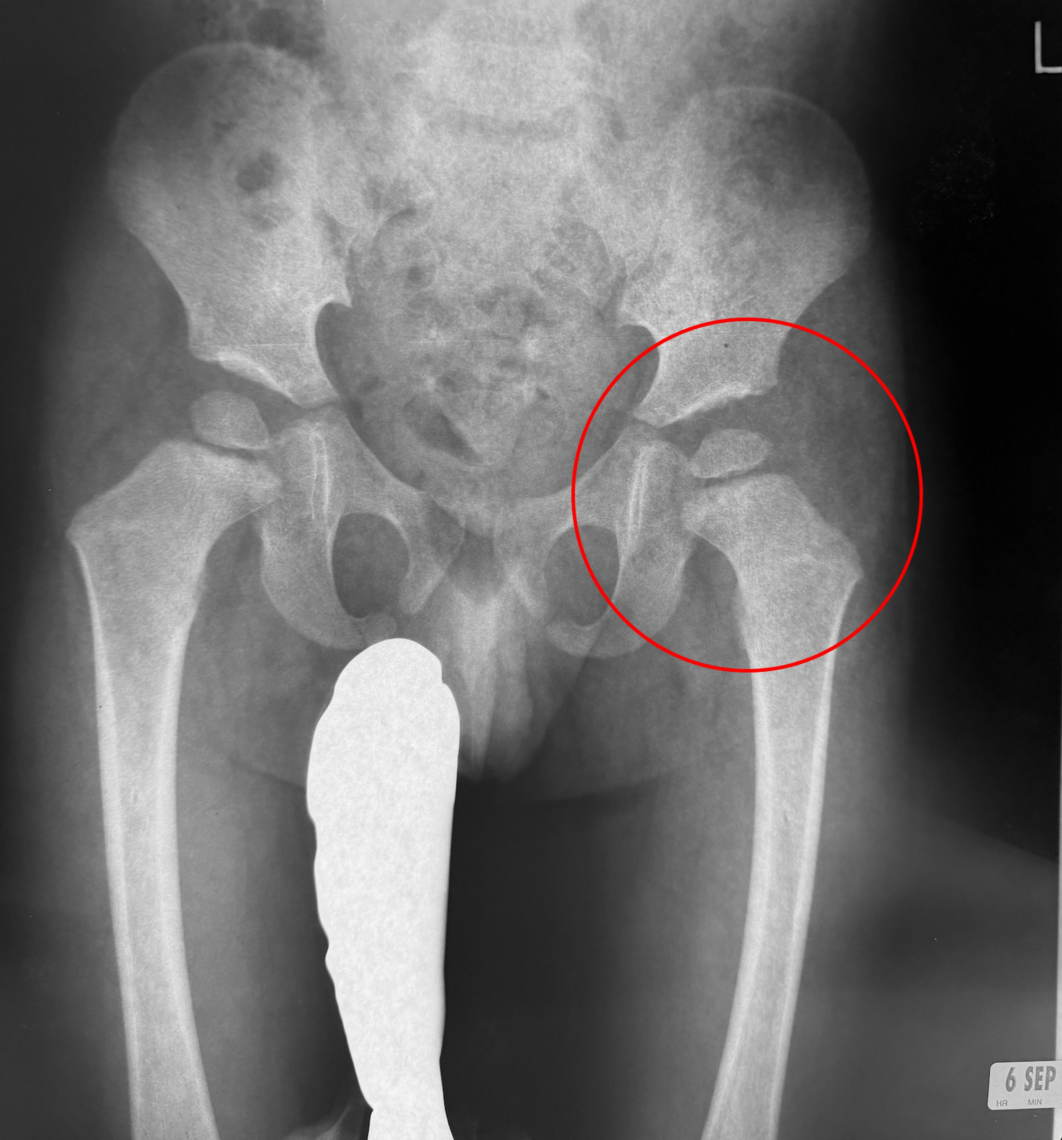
Supine anteroposterior pelvic X-ray taken on September 6, 2005.
